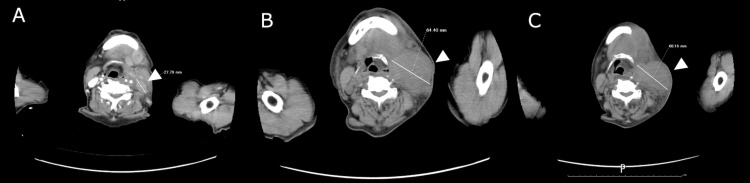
Serial computed tomography scans of cervical lymph node metastases Serial computed tomography scans of cervical lymph node metastases (white triangles): (A) at recurrence diagnosis in May 2016, (B) before radiotherapy in August 2017, (C) at the time of adopting best supportive care in February 2018.
